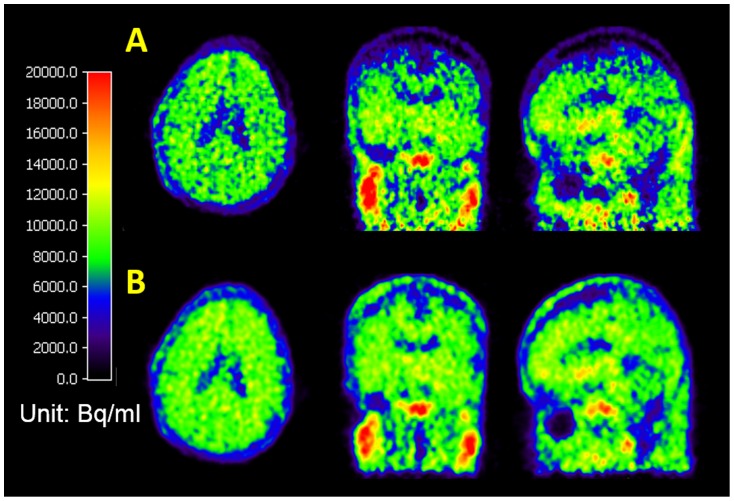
Improvement of a late FDDNP PET/CT frame image after MC. Panel A: An FDDNP PET/CT study on a Down syndrome patient with 10 mm movement in z direction in a late frame of a dynamic scan. Panel B: 3-D orthogonal images show the improvement of the PET/CT images with proper AC after MC. All images are displayed with the same color scale and show clearly the quantitative differences in the PET images reconstructed with misaligned and aligned CT images.
